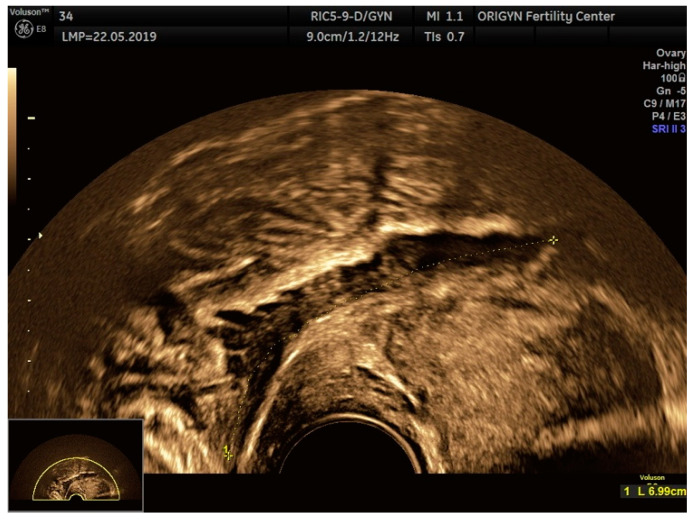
Ultrasound image showing a big DIE nodule with some spikes towards the bowel lumen, in extrinsic retraction. In this case, the sliding sign was absent.
